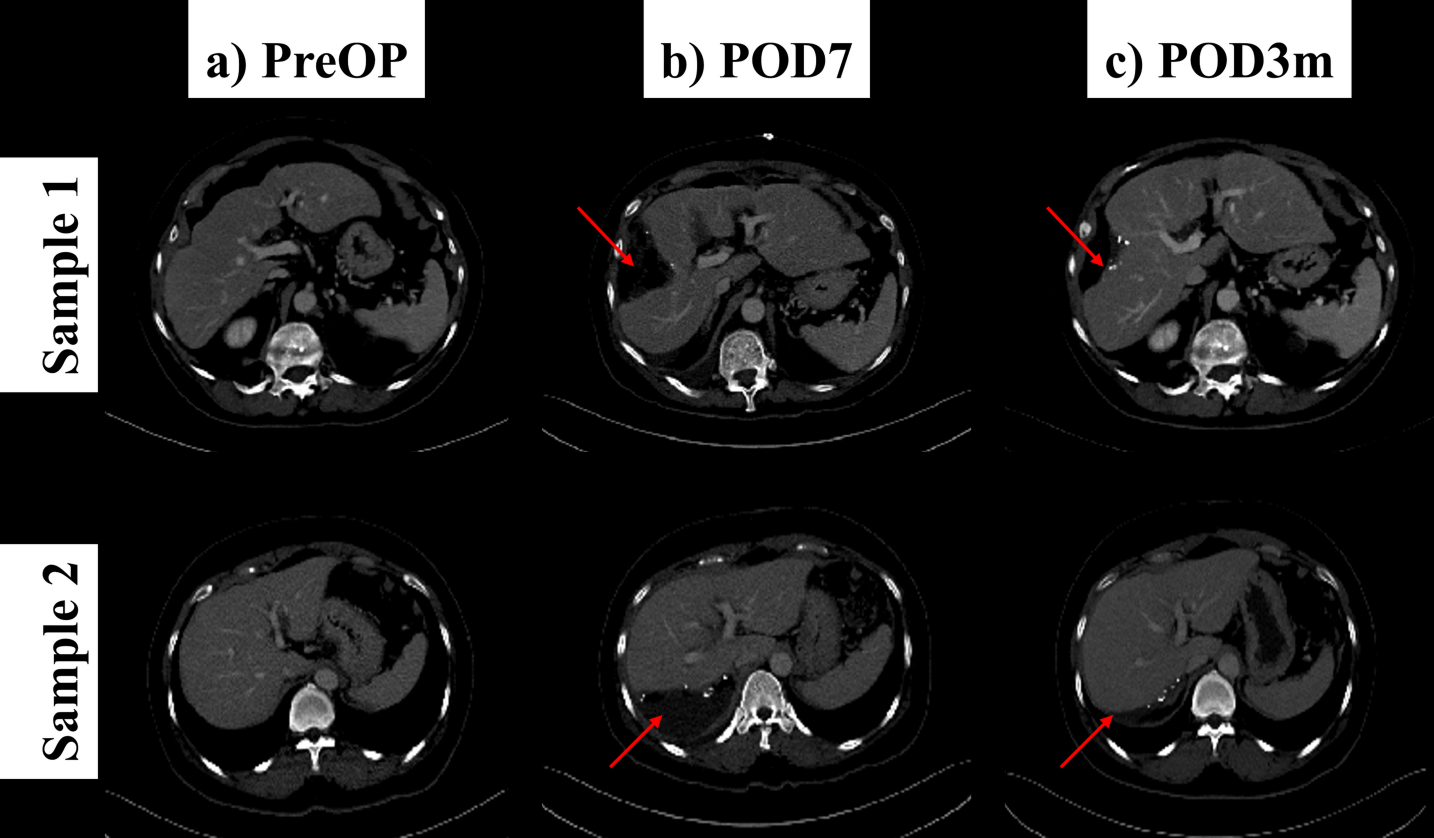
This figure depicts representative axial CT images demonstrating liver volume changes (Red arrows) following hepatectomy from two different patients (Samples 1 and 2) at three distinct time points: (a) Pre-operative (PreOP), (b) 7 days post-operative (POD7), and (c) 3 months post-operative (POD3mo).
